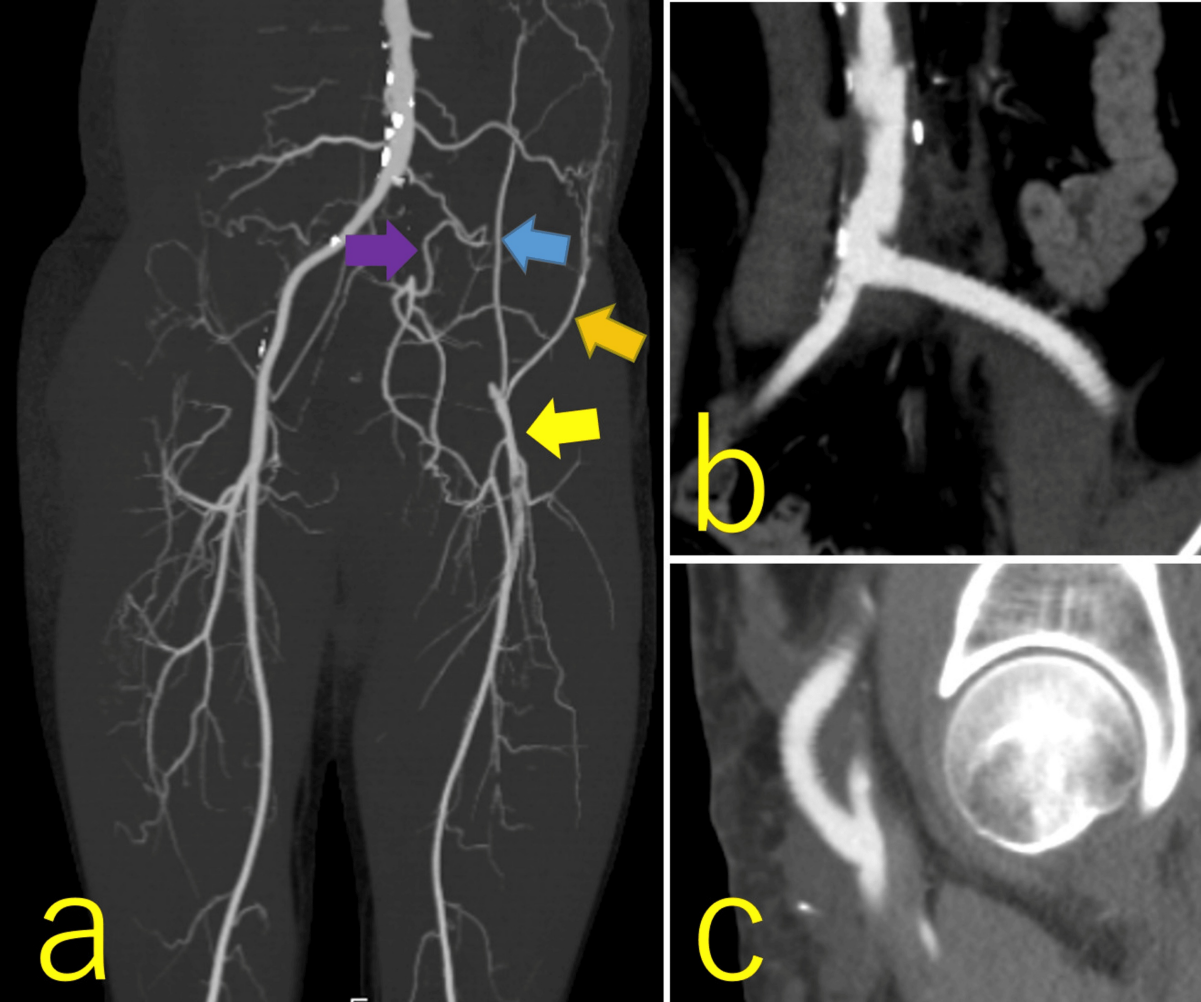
Pre- and postoperative computed tomography (CT) findings of the prosthetic bypass between the terminal aorta and left common femoral artery (CFA) a: occlusion from the left common iliac artery to the CFA, and developed collateral arteries, including the left lumbar artery (purple arrow), inferior epigastric artery (blue arrow), and circumflex artery (orange arrow), which drained into the left CFA (yellow arrow); b: proximal anastomosis site of the prosthetic bypass between the terminal aorta and left CFA; c: distal anastomosis site of the prosthetic bypass between the terminal aorta and left CFA.
